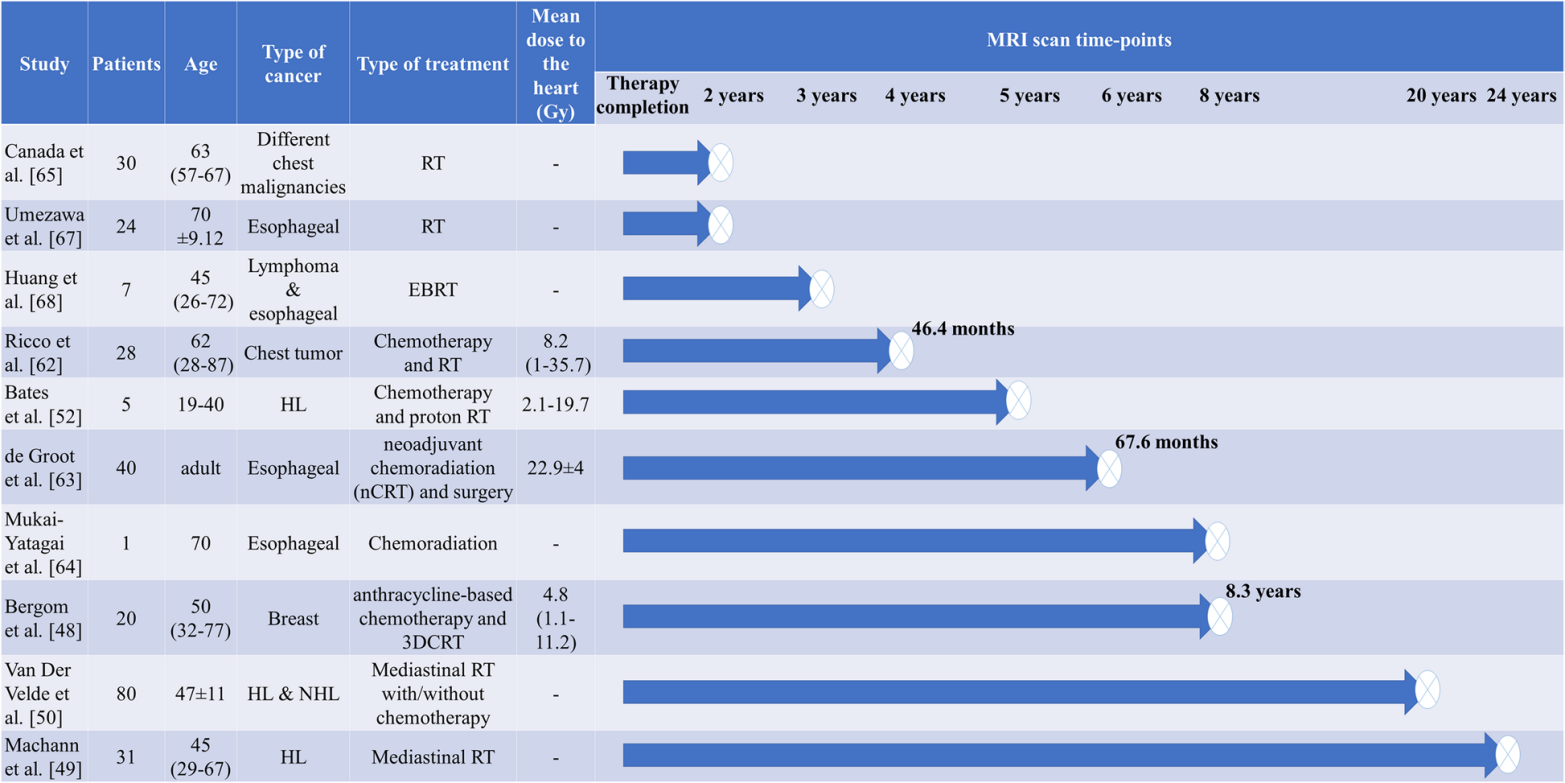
Details of cross-sectional studies including number of patients, age, type of cancer, type of cancer treatment, radiation dose to the heart and timing of MRI measurements
